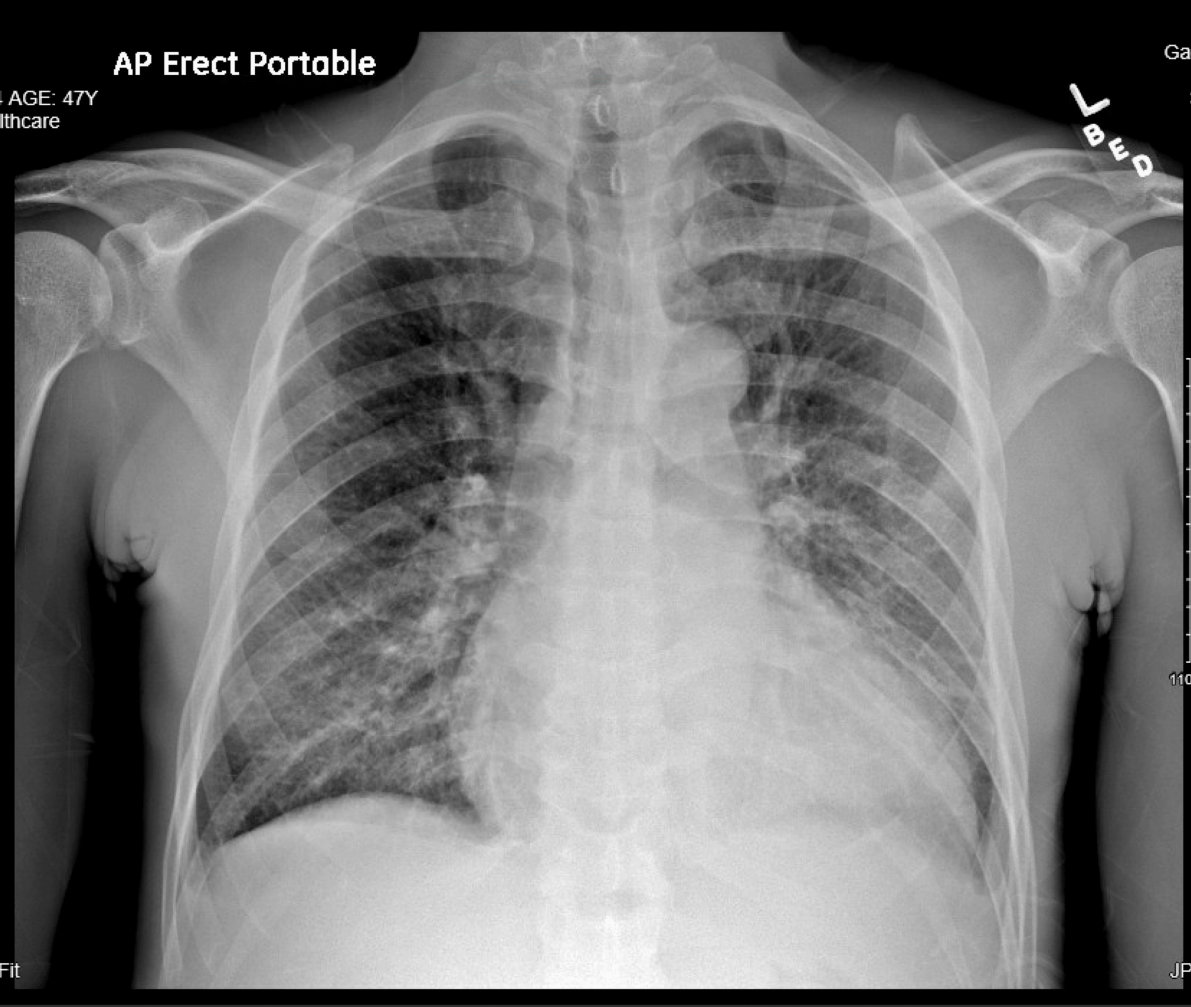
Chest radiograph, in an anteroposterior view. Enlarged cardiac silhouette, accompanied by bilateral increased interstitial markings and cephalization of pulmonary vessels, indicative of pulmonary vascular congestion.
